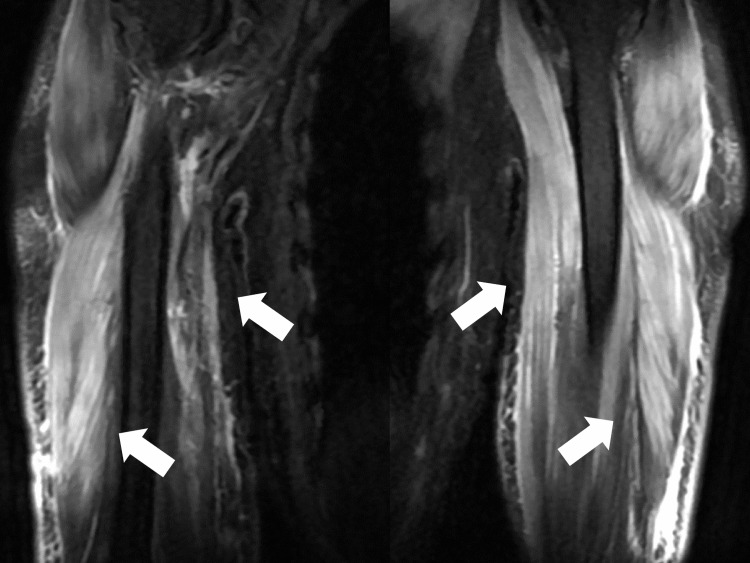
Magnetic resonance imaging (fat suppression images) of both arms shows high signal in the entire muscles of both arms (white arrows)
